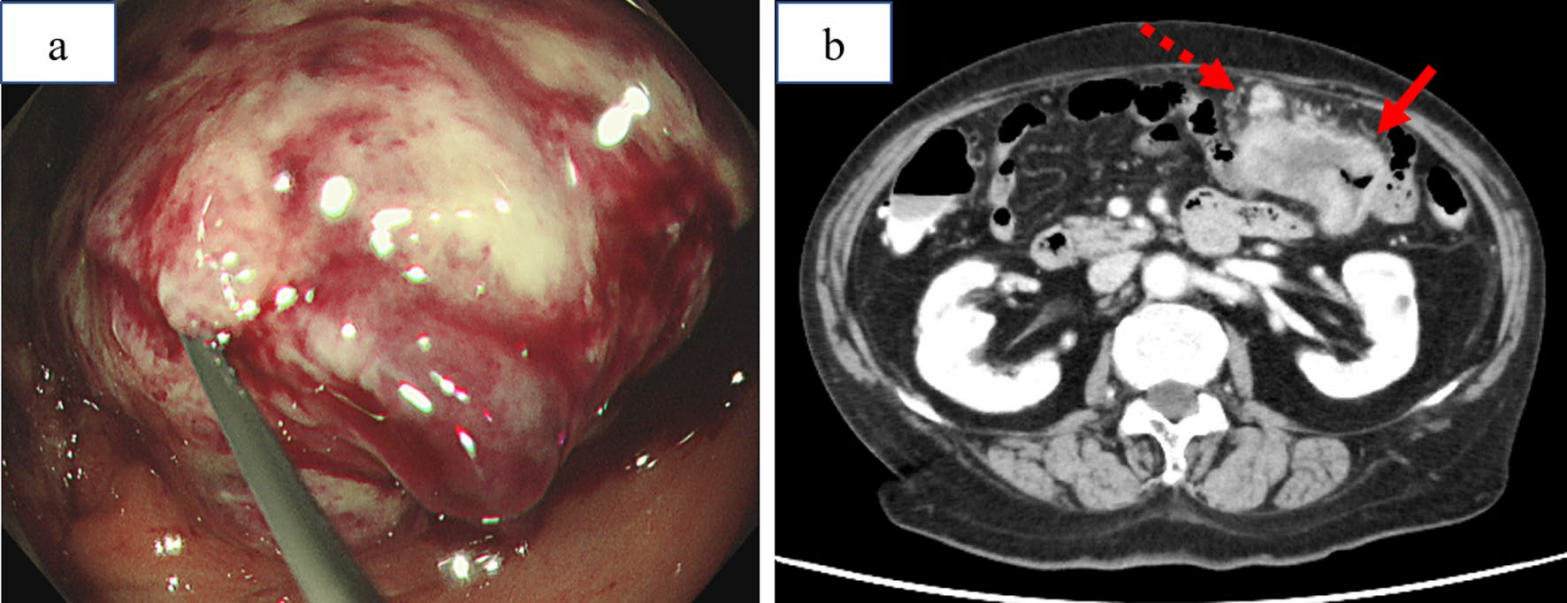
Preoperative examinations. a Colonoscopy confirmed an obstructive tumor in the transverse colon. b Computed tomography scan showed thickening of the transverse colon (solid arrow) with enlarged lymph nodes (dashed arrow)
